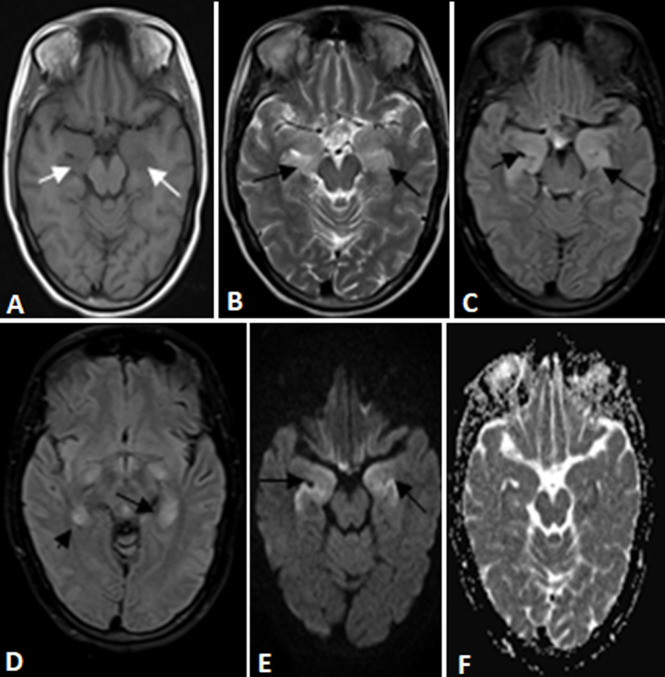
magnetic resonance imaging of brain showing hypointensity over bilateral medial temporal lobes on T1 weighted images (panel A marked with white arrows); hyperintensity over bilateral medial temporal region on T2 weighted (panel B); FLAIR sequences (panel C and D marked with black arrows) with no diffusion restriction; (panel E, diffusion weighted images and F, apparent diffusion coefficient images)
